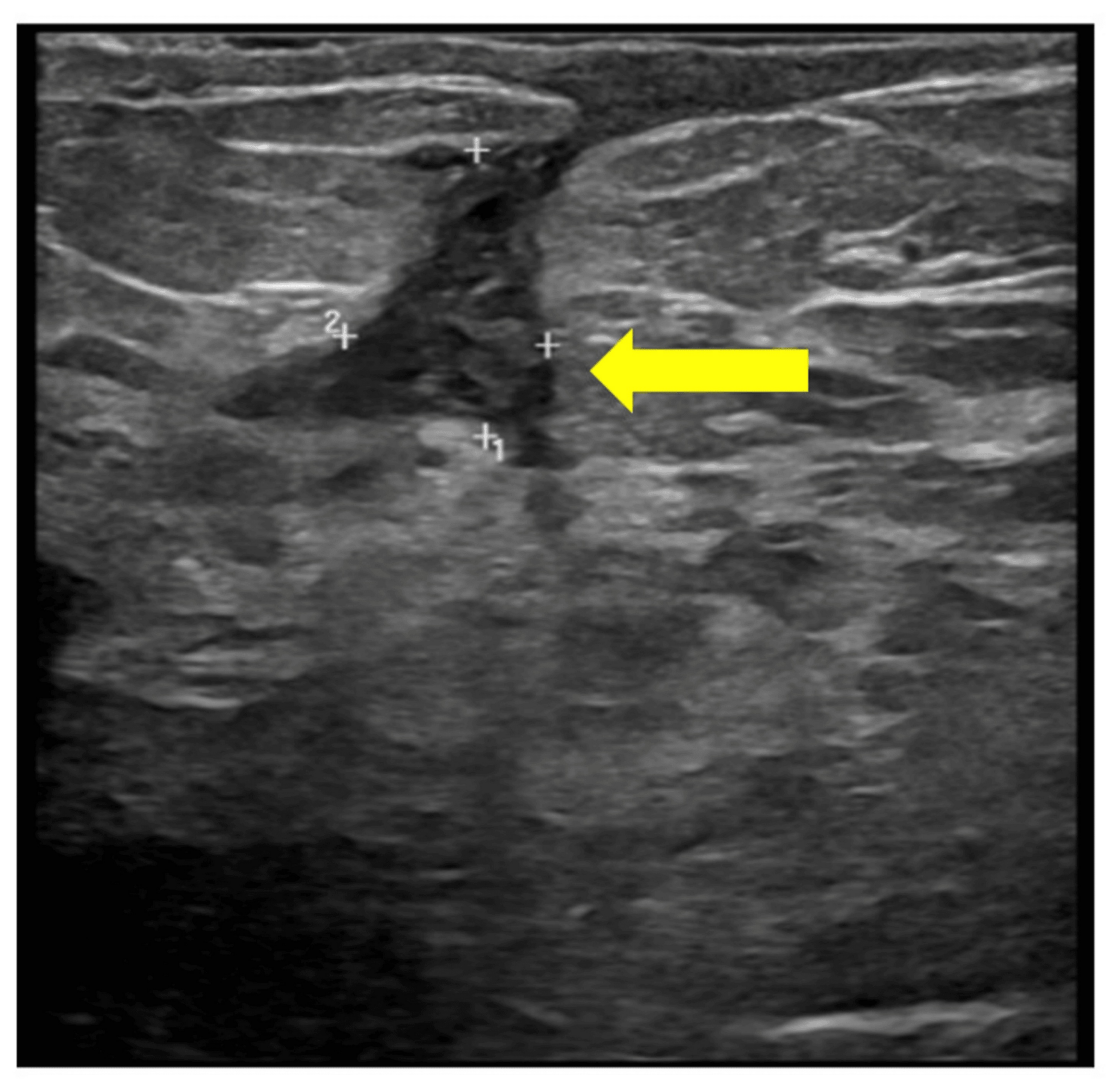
Bilateral breast ultrasound: diffuse edema in the left breast with thickened sinus-like formation (arrow).
